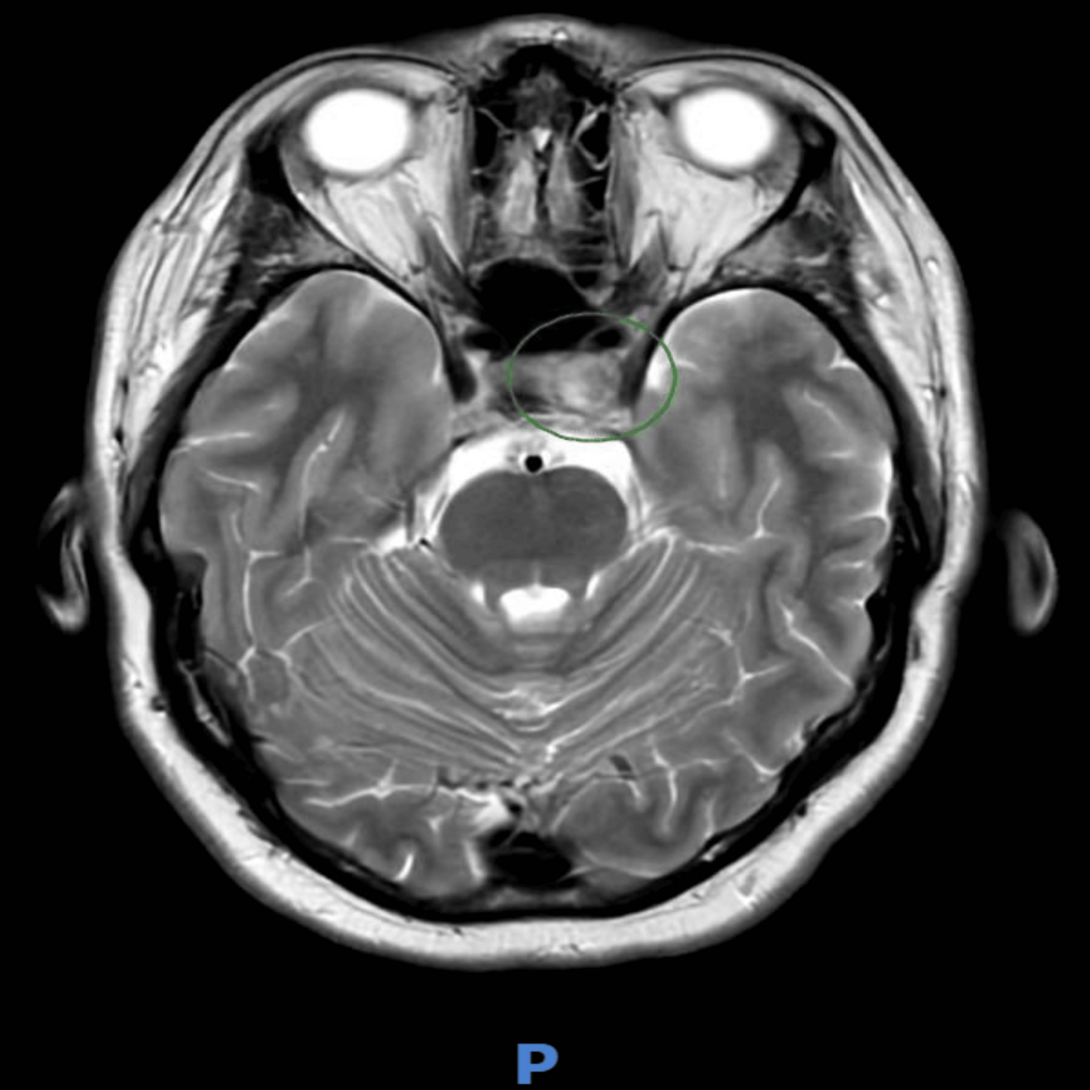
Pituitary MRI with contrast imaging. Asymmetrical enlargement of the left pituitary gland, which had a cranial-caudal thickness of 1 cm. Slight suprasellar extension, with midline pituitary stalk and no compression of the optic nerve/chiasm. On pre-contrast images, there was an abnormally high T1-weighted signal in the left lobe. On post-contrast images, there was a 1 x 1.2 x 1 cm corresponding area of reduced enhancement in the left loop, with a suggestion of partial encroachment onto the left cavernous sinus.
